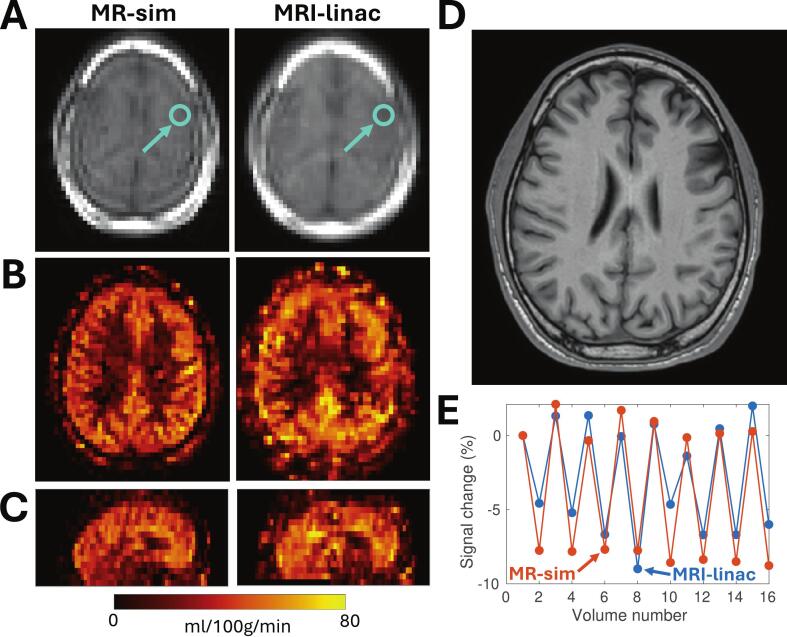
Comparison of MRI-linac and MR-sim ASL in the same healthy volunteer. (A): Axial views of the control acquisition for ASL in one healthy volunteer scanned on both the MRI-linac and MR-sim. (B, C): The corresponding CBF maps in an axial and sagittal view. (D): A T_1_-weighted image in the same slice from the MR-sim is shown for anatomical reference. (E): The average signal versus volume number over an 8 × 8 × 8 mm^3^ region of interest in grey matter (cyan regions in A). The volumes alternate between control and label acquisitions, repeating for eight dynamics. The signal is normalized to the first volume for each scanner. The magnitude of the signal fluctuations is slightly smaller for the MRI-linac (6.7 ± 1.5 percentage points) compared to the MR-sim (8.7 ± 0.9 percentage points), consistent with the lower labelling efficiency (Fig. 2). (For interpretation of the references to color in this figure legend, the reader is referred to the web version of this article.)
